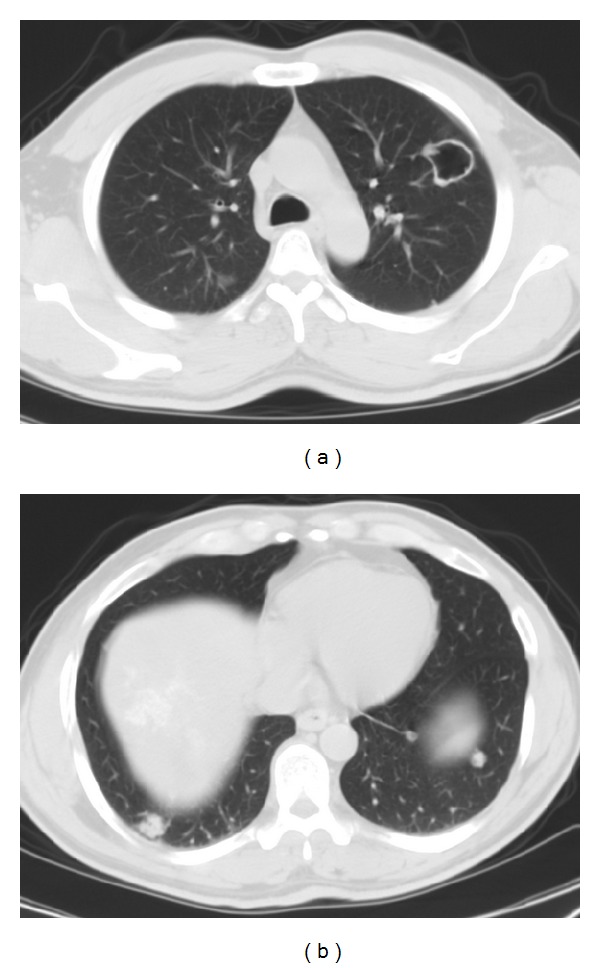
Thorax CT reveals multiple noncalcified nodular lesions in both lungs (a). A cavitary lesion with thick irregular wall is seen in the upper lobe of the left lung (b).
